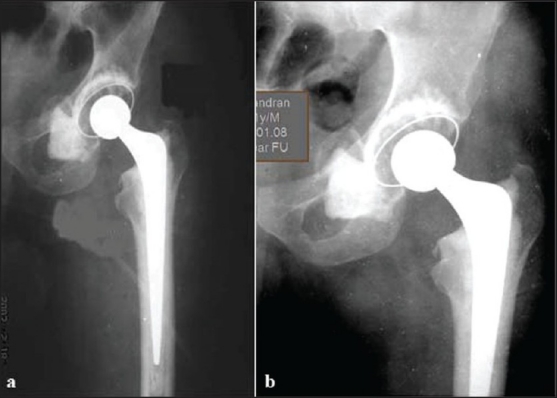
Immediate postoperative (a) and one year followup (b) X-ray of left hip showing cemented total hip arthroplasty
